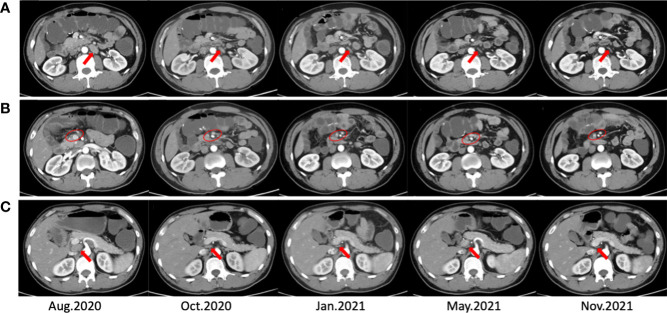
CT scans before, during, and after the PRaG therapy. The CT scans (A, B) showed shrunk and disappeared of irradiated lymph node metastases. The CT scans (C) showed shrunk and disappeared of nonirradiated lymph node metastases. The arrows point to individual lymph nodes, and the circles include fusion lymph nodes.
